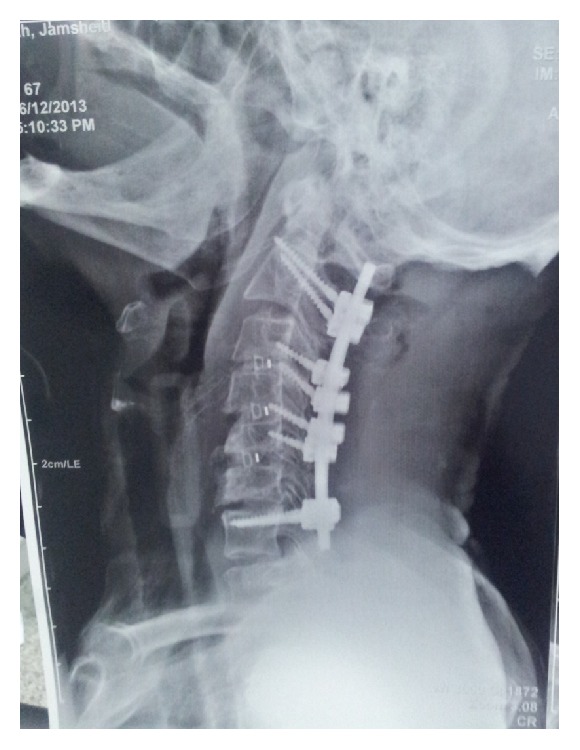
Postoperative lateral cervical radiograph, 3-level ACDF, and C2 to C7 pedicle screw resulting in optimal correction of the deformity.
